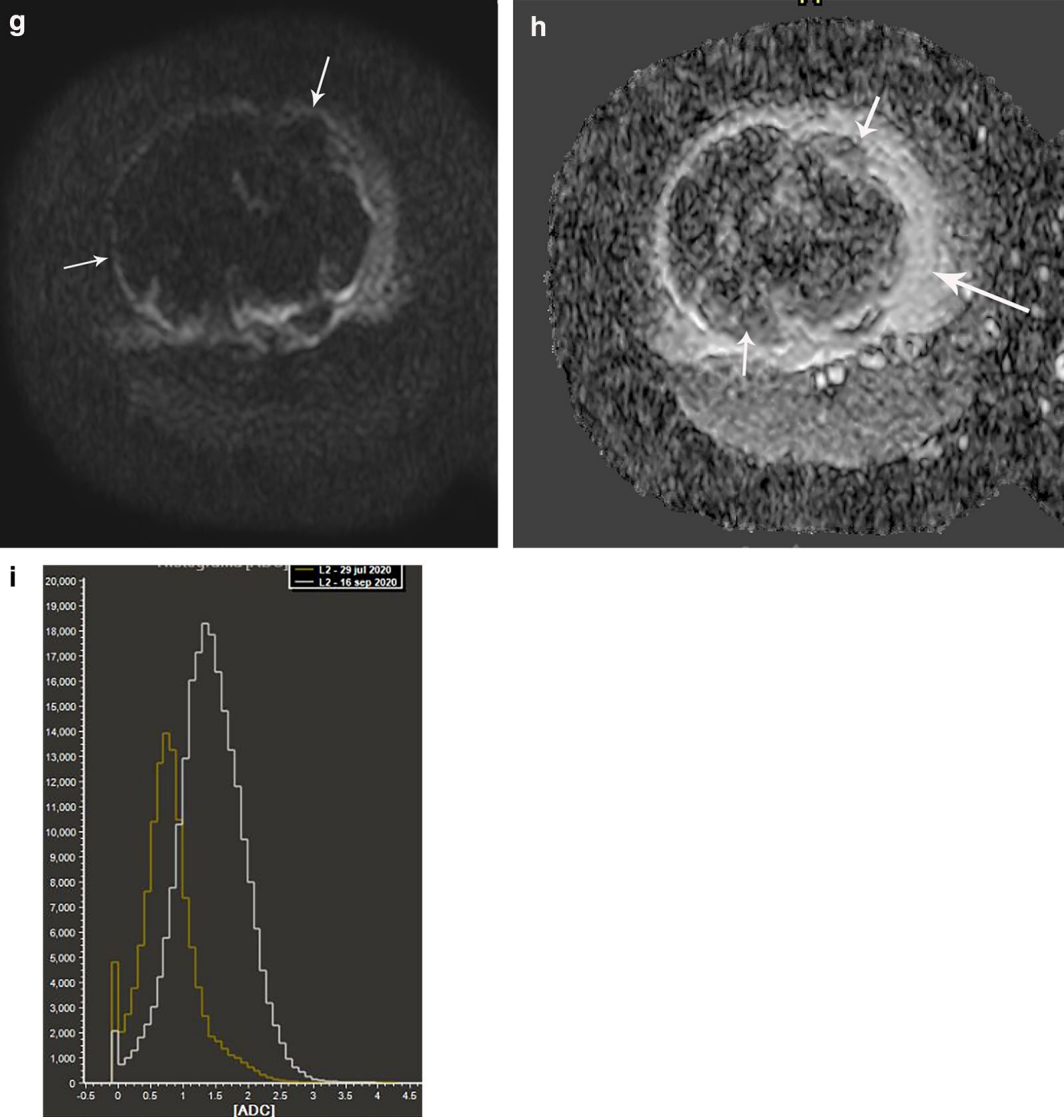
Ewing sarcoma of the distal femur in a 9-year-old boy. a-d) Pre-treatment. a Axial contrast-enhanced fat-suppressed T1-weighted MR image before treatment shows a large tumor heterogeneously enhancing (short arrows) with internal necrosis (*). b Automated volumetry of the mass is around 285 cc. c and d Axial DWI and ADC map images show restricted diffusion in the periphery of the lesion (arrows). Post-treatment. e Axial contrast-enhanced fat-suppressed T1-weighted MR image after treatment shows increase of the internal necrosis of the mass (*) with persisting peripheral enhancement (short arrows). Note soft tissue inflammation not present in the previous MRI (long arrow). f Automated volumetry of the mass is around 350 cc. g and h Axial DWI and ADC map images show mild linear restricted diffusion of the periphery (arrows). i Histogram of ADC maps performed before and after treatment shows an increase of the ADC mean values from 0.79 mm2/s to 1.41 mm2/s
